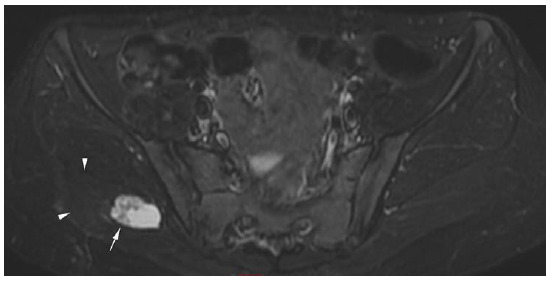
Axial MRI pelvis – complete resolution of soft tissue oedema (arrowheads) surrounding the mature lesion (arrow). Central soft tissue T2 hyperintense and T1 isointense. Axial measurement is 3.1 x 2cm.
